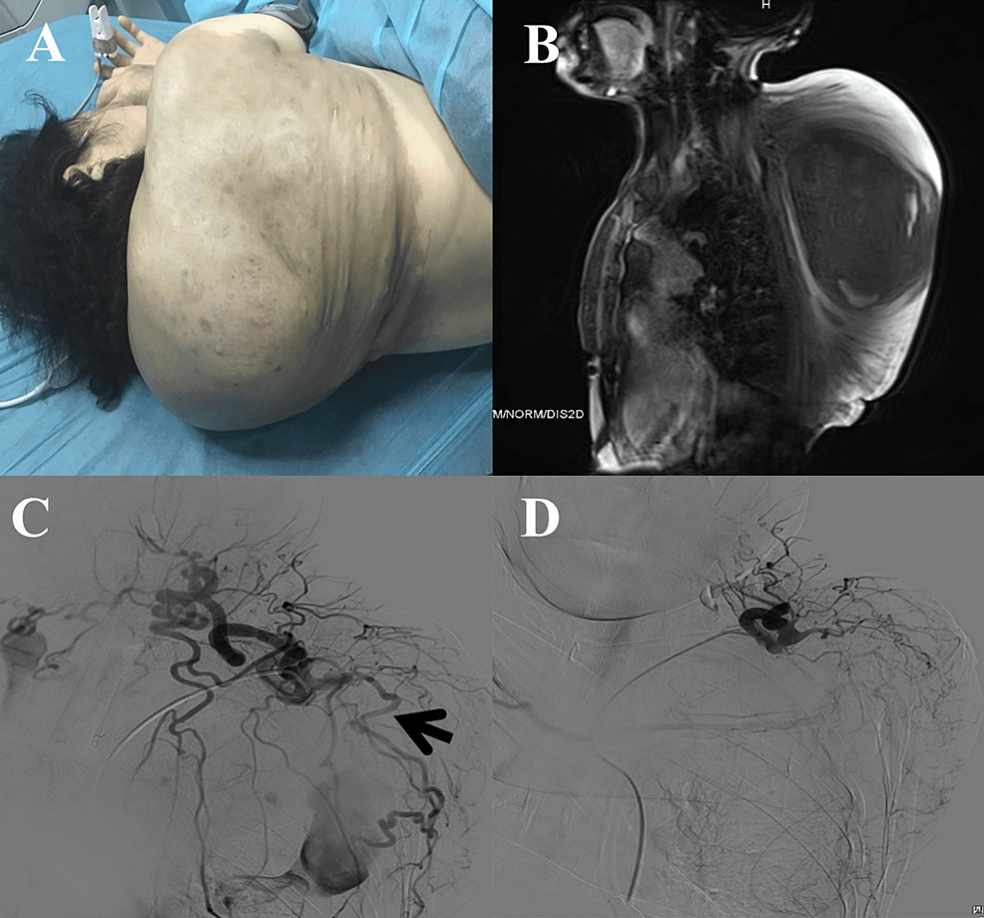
Malformed tumor vessel bleeding treated by interventional embolization in a NF1 patient A: a female NF1 patient presented with a massive back hematoma after acupuncture; B: MRI showing a massive back hematoma (mid-sagittal plane); C: subclavian artery angiography revealed multiple enlarged and tortuous arteries (arrow); D: the culprit artery was embolized MRI: magnetic resonance imaging; NF1: neurofibromatosis type 1
